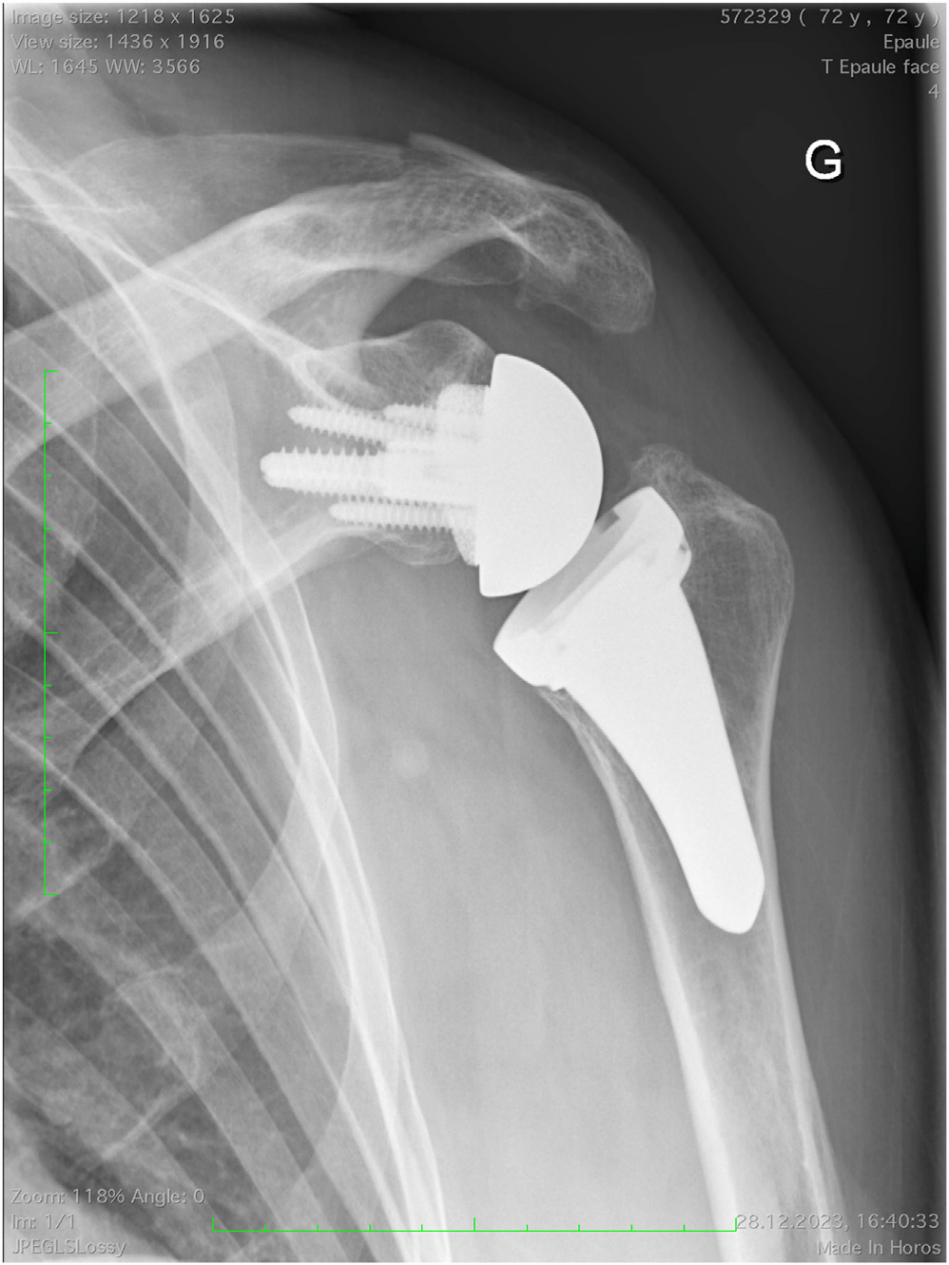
Anteroposterior x-ray of a left shoulder. A reverse shoulder arthroplasty had been implanted 3 years previously with satisfactory results according to the patient. He sustained an ipsilateral traumatic scapular spine fracture in a skiing accident.
